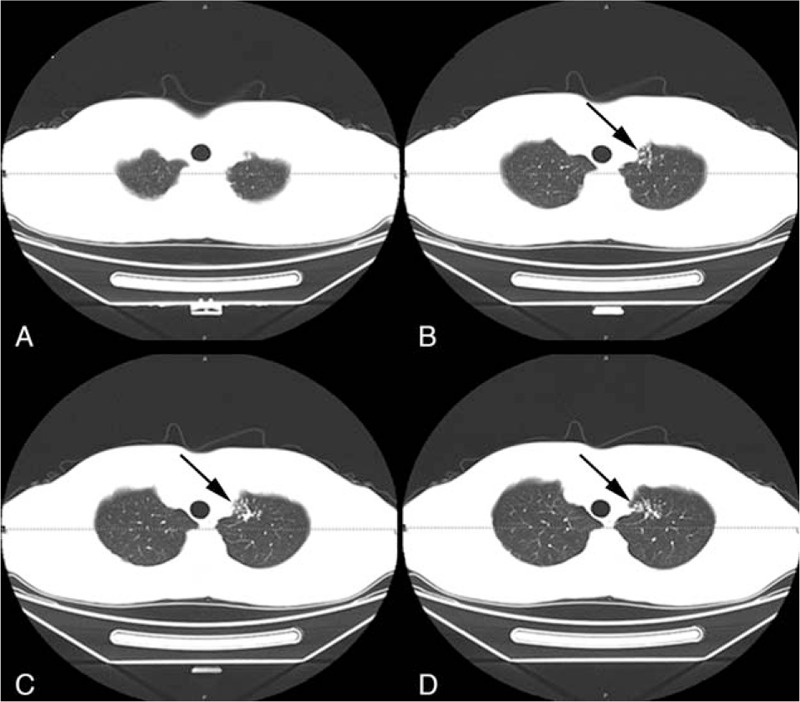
The CT scanning of the lung. (A) There is no real change in the lung field. (B, C, D) CT scanning showed some point patchy shadows in the left apex pulmonis (black arrow). CT = computed tomography.
